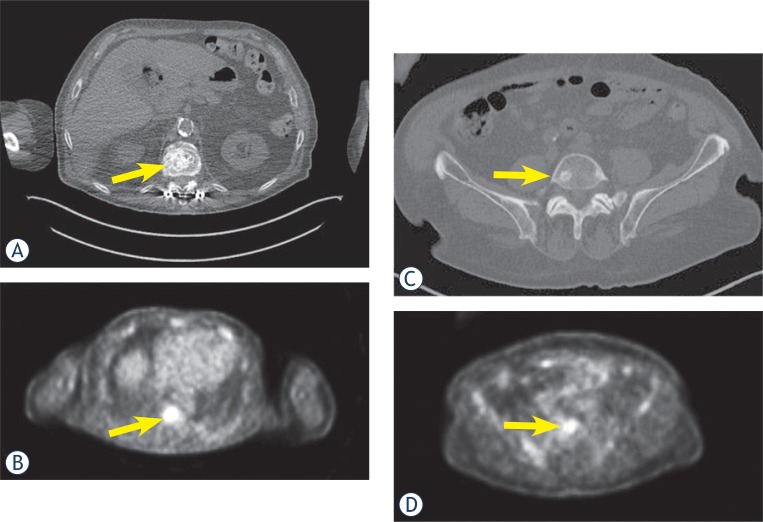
A and C. CT scan shows osteoblastic metastases in lumbar vertebral bodies (arrows); B and D. the same lesions show metabolic activity on PET-scan (arrows).
